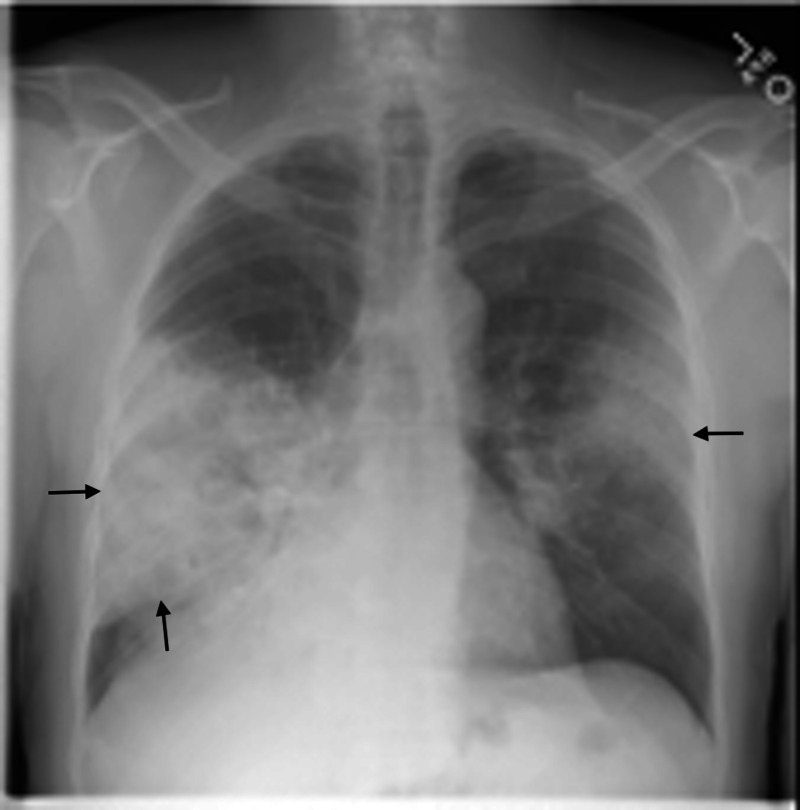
Chest X-ray four months prior to diagnosis Arrows indicate areas of focal consolidation involving the right middle lobe, portions of the right lower lobe, and left midlung
